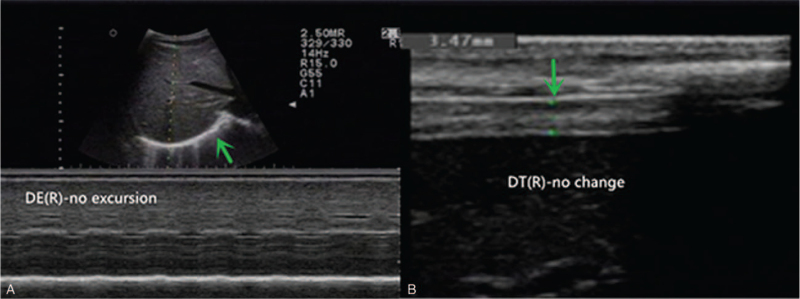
Ultrasonographic examination of the right diaphragm. The hyperechoic window of the right diaphragm in the 7th costal space could be observed at the distal end of the liver. A, The diaphragm excursion (DE) was observed by M-type ultrasound with no observed diaphragm movement (green arrow). B, The right diaphragm thickness (DT) (3.5 mm) was observed through B-type ultrasound, with no change during inspiration (green arrow).
